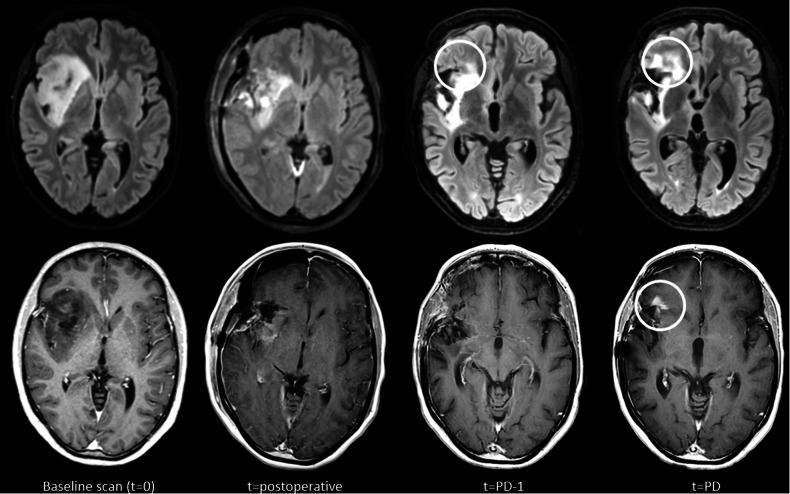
Radiological follow-up of an exemplary patient, showing increased enhancement on the CET1w image and increased hyperintensity on the T2w-FLAIR image at the time of progression (PD), as well as earlier increased abnormalities on the T2w-FLAIR image preceding new contrast enhancement
